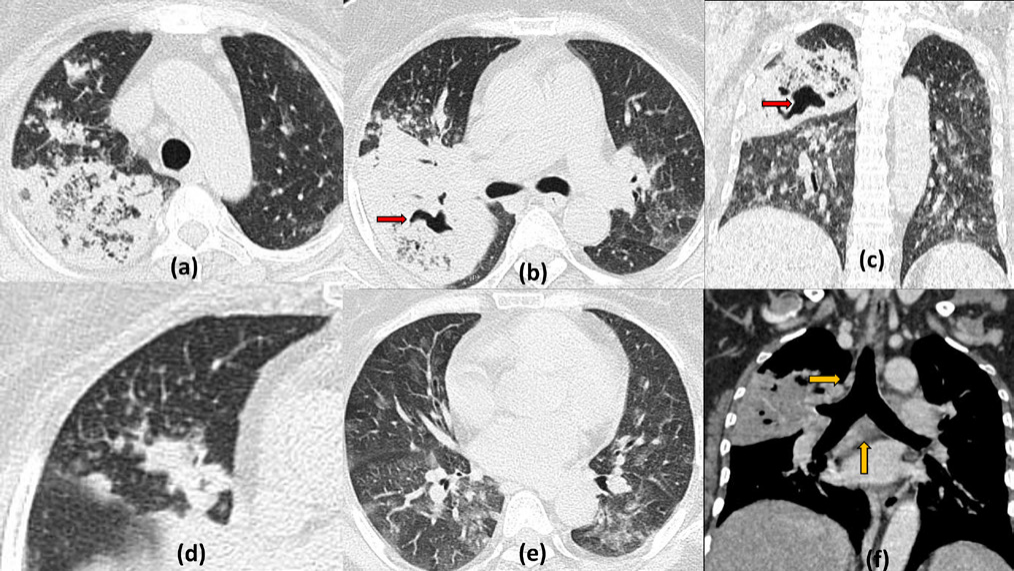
Active cavitating *Mycobacterium tuberculosis* coinfection with COVID19: 46-year-old female presented with high grade fever and acute shortness of breath and tested positive for COVID19 infection. Contrast enhanced CT lung window sections of the chest (a–e) revealed right upper lobe consolidation with central cavitation (red arrow) with multiple centrilobular nodules, some showing tree in bud appearance (zoomed image d). Multifocal rounded ground glass opacities were also seen in the bilateral lower lobes due to Covid19 pneumonitis. Coronal mediastinal window (f) shows heterogeneously enhancing subcarinal and paratracheal mediastinal lymph nodes. Sputum tested positive for Acid Fast Bacillus confirming *Mycobacterial* coinfection.
